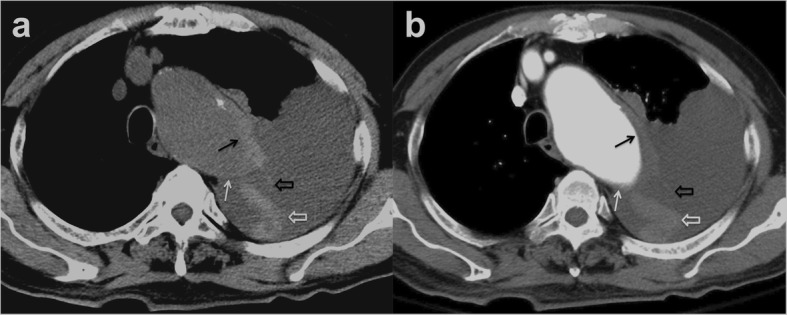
a Unenhanced axial CT shows a smooth eccentric defect (white arrow) in the hyper-attenuating crescentic hematoma (black arrow) along the descending thoracic aorta and (b) corresponding focal out-bulging (white arrow) of the aortic lumen with smooth obtuse edges in enhanced study, outward displacement of partially torn adventitia (open black arrow), dislodged blood clot (white open arrow), and left pleural effusion. Note the absence of intimal flap or contrast medium extravasation
